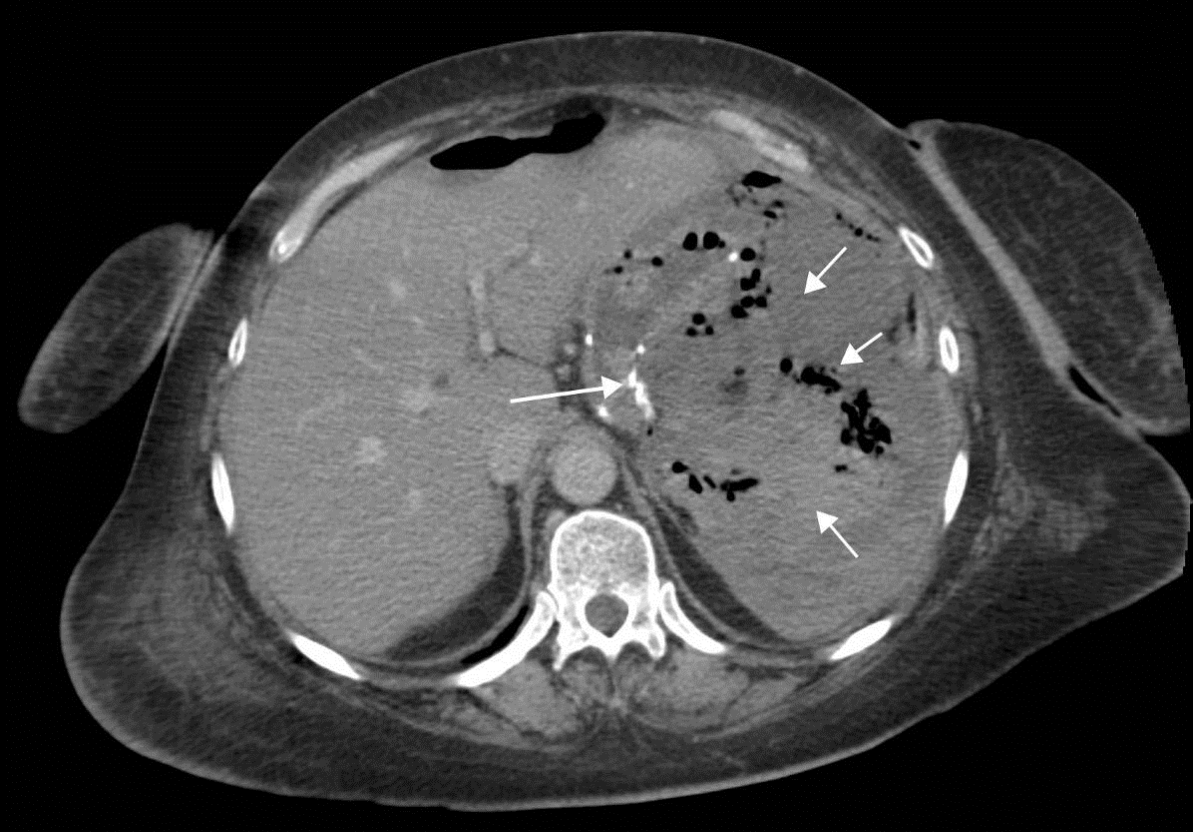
Upper abdominal CT scan with IV contrast enhancement 10 days post bypass procedure, showing a large irregular abscess containing gas and semi-solid material in the upper abdomen (short arrows). Note the upper margin of the gastric staple line (long arrow). The collection was drained percutaneously but a further laparotomy was required to repair a leak at the gastro-jejunostomy site.
